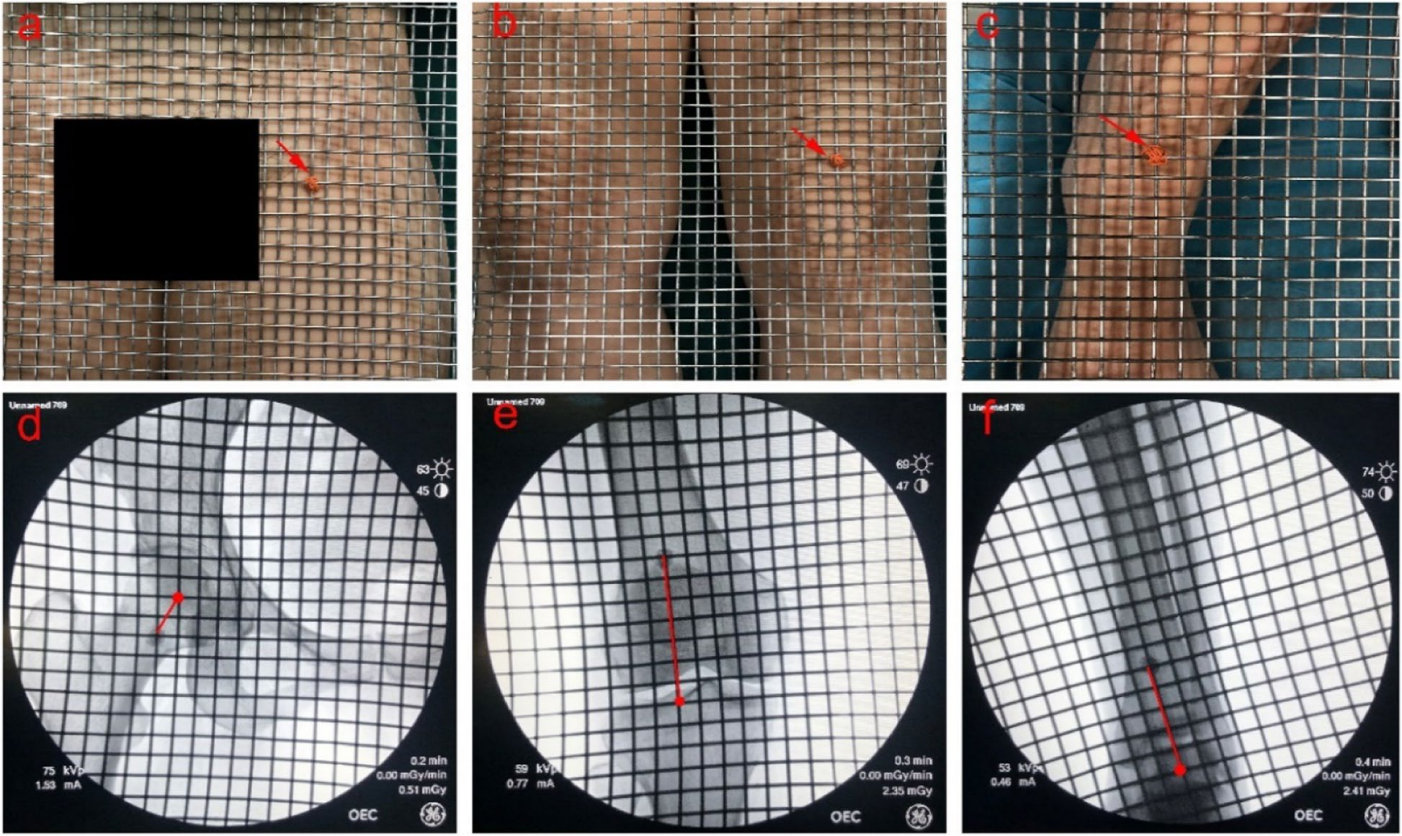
a~c An orange coil (arrow) was used as a reference point. d~f The hip centre, knee (Fujisawa point), and ankle centre (red points) determined using X-ray fluoroscopy. The red line represents the distance from the reference point (orange coil) to the target points. This method can save time and allow the projection of the hip centre, Fujisawa point, and ankle centre to be located more easily
